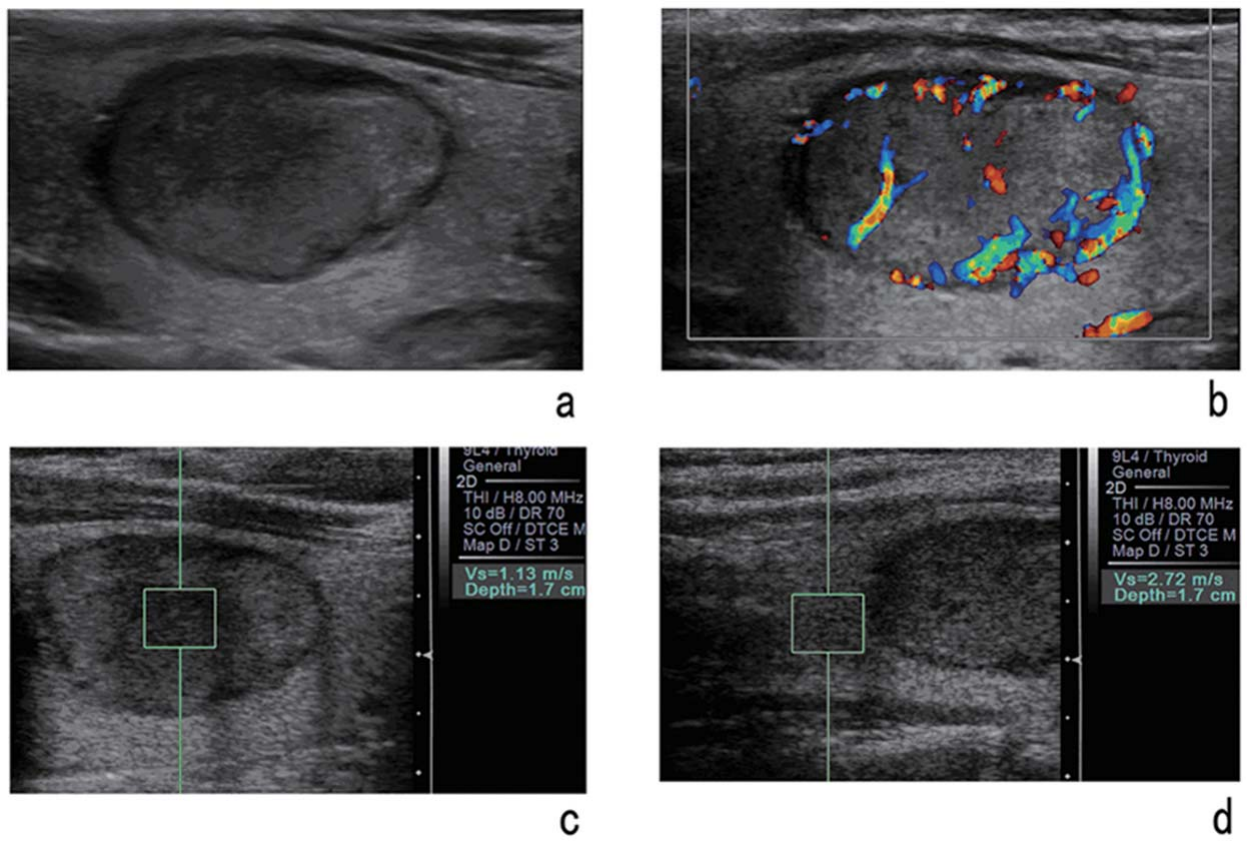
A 42-year-old woman with a solid lesion of nodular goiter. Gray-scale image (a) and color Doppler image (b) of the nodule. VTQ of the nodule (c) shows the SWV is 1.13 m/s and the SWV of the adjacent thyroid tissue (d) is 2.72 m/s.
